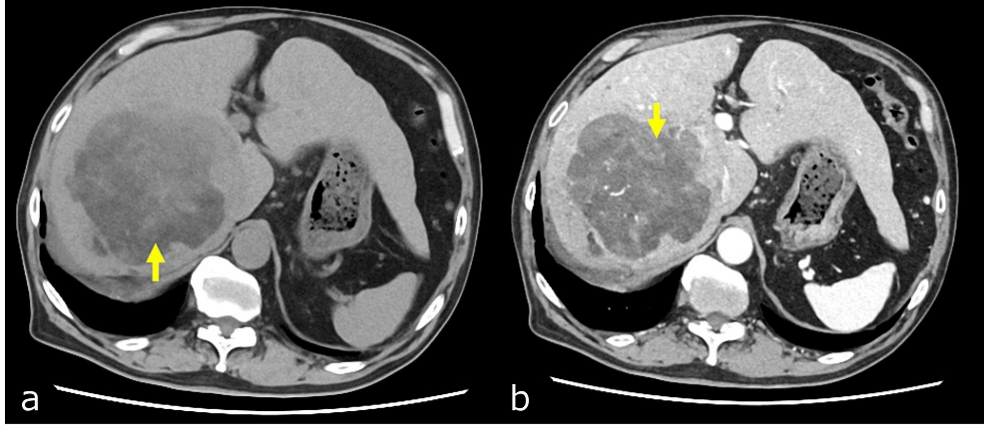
Abdominal CT scan Abdominal CT shows a 12 cm mass lesion in the posterior segment of the right hepatic lobe. The interior of the mass exhibits lower attenuation values than the liver parenchyma (a, indicated by yellow arrows). On contrast-enhanced CT, numerous blood vessels are observed internally, and early enhancement is clearly visible at the tumor margins (b, indicated by yellow arrows).
